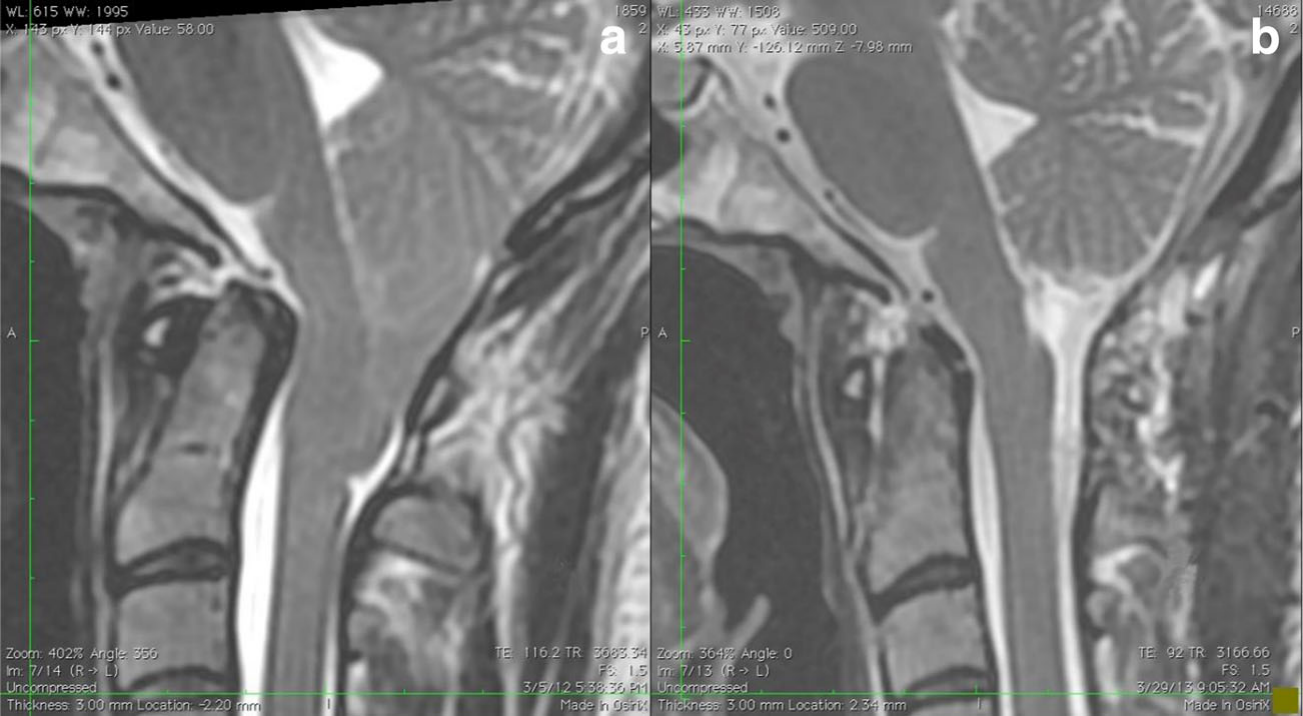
a Preoperative and b postoperative MRI of a MIST procedure and it should be noted this patient concomitantly underwent a craniocervical fusion for basilar invagination
